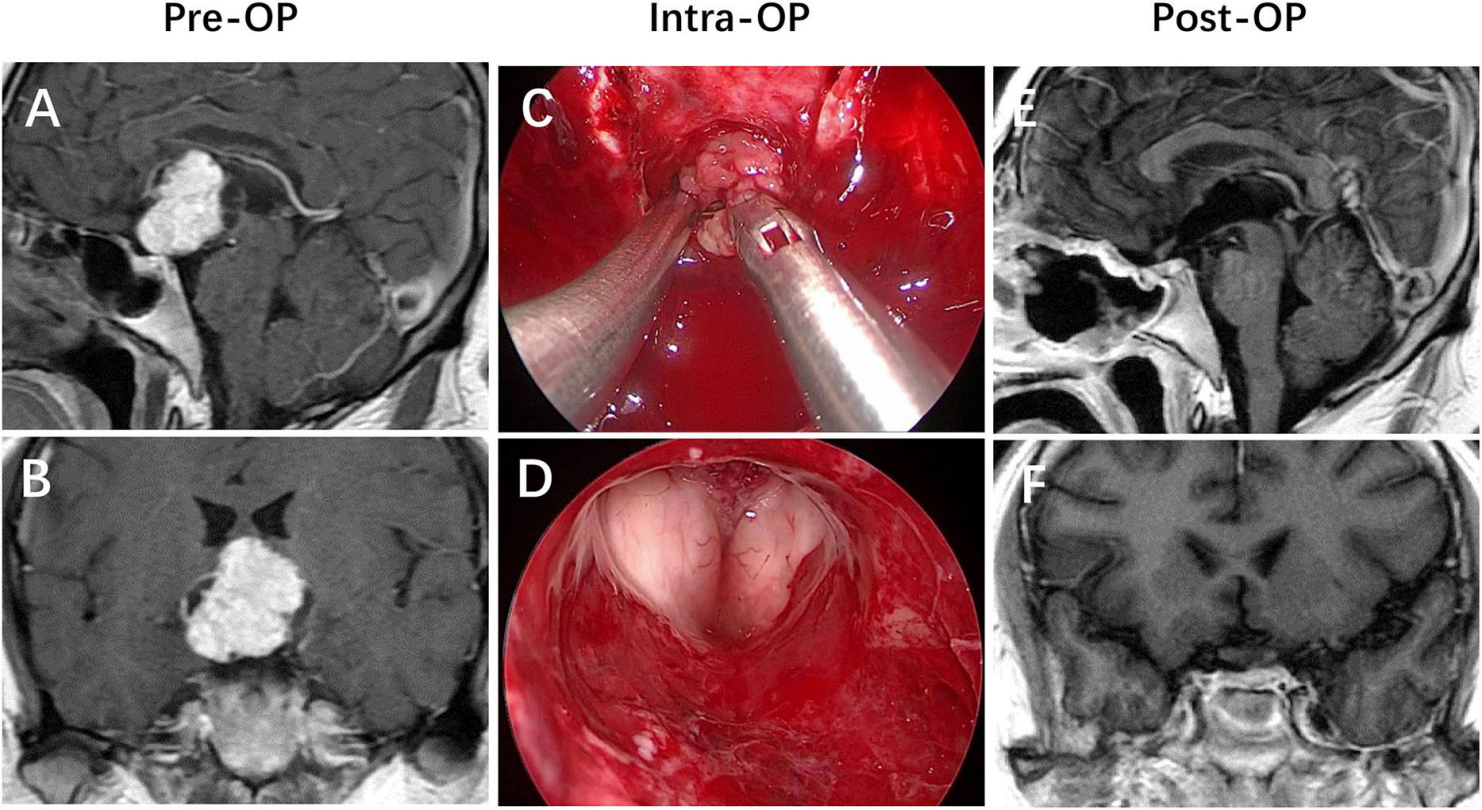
Not strictly intraventricular craniopharyngioma. (A,B) Preoperative enhanced MRI demonstrated that a mass had primarily developed within the neural tissue of the third ventricle floor (TVF), progressively replacing part of the floor while expanding into the third ventricle cavity, in which expansion of the tumor has breached the TVF. (C,D) Intraoperative views revealed that the patient achieved a gross-total resection of the lesion *via* the endonasal endoscopic endonasal infrachiasmatic corridor. (E,F) Postoperative enhanced MRI revealed that the lesion was totally removed, with the posterior wall of the third ventricle and the pituitary stalk preserved intactly.
